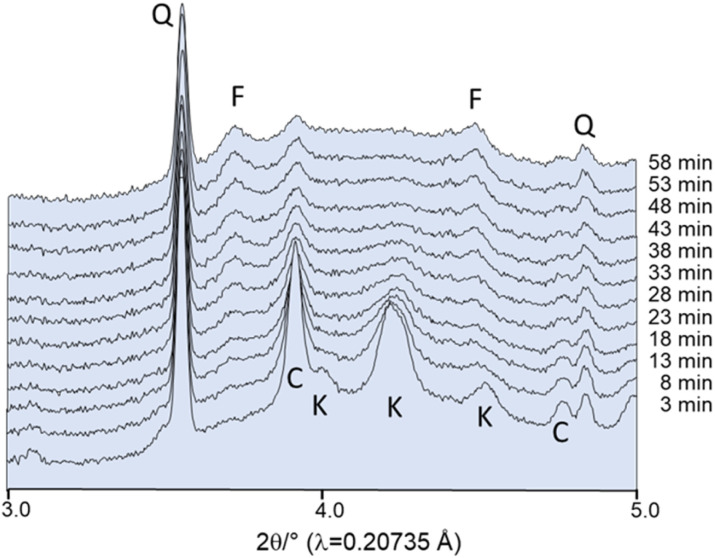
In situ* X-ray powder pattern collected during milling at 25 Hz: F: fairchildite, C: CaCO_3_, K: K_2_CO_3_, Q: quartz. Intensities are plotted on a square root scale with an offset between the datasets.
